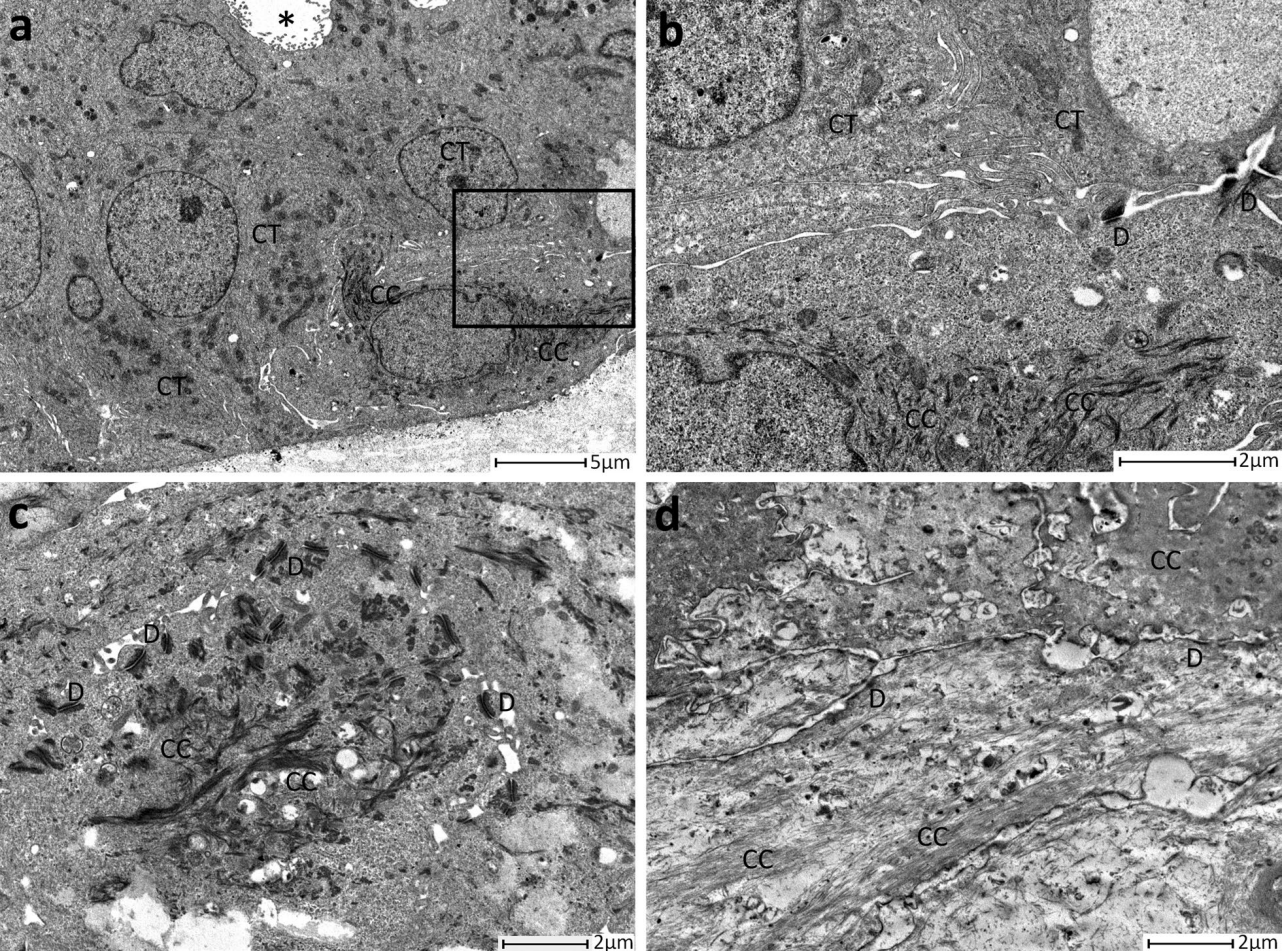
a Representative image of a single cell with numerous keratin (CC) filaments. This cell was in basal position and in closed contact with tumour cells (CT). b Magnification of panel a that depicted one cell in basal position, full of keratin (CC) filaments. Also, the neighbouring tumour cells (CT) were displayed. c Magnification of keratin (CC) filaments. d Representative image of keratinized cells. The cytoplasm is full of keratin filaments (CC), “ghost” desmosomes and cellular organelles were absent. Intercellular lumen = *, D = desmosome
